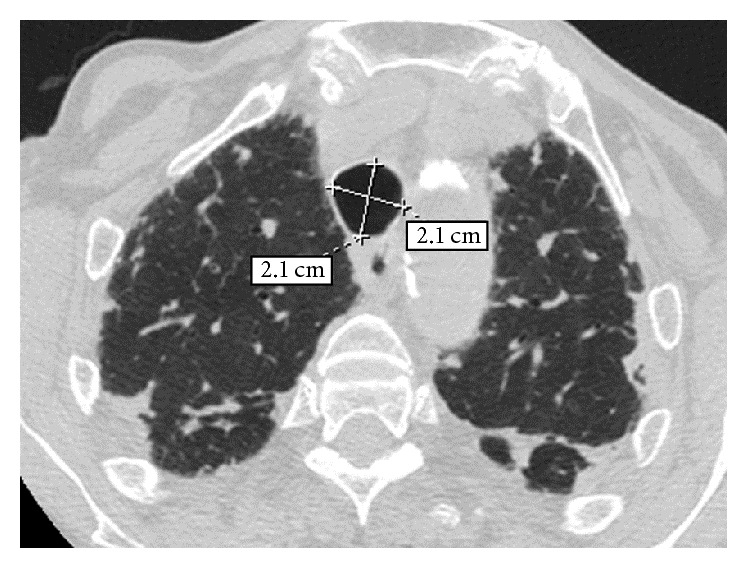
High-resolution computed tomography (HRCT) of the chest at the level of the upper lobes. An enlargement of the trachea at the thoracic inlet is observed measuring in both sagittal and axial dimensions 2.1 cm. A subpleural reticular pattern associated with focal thickening of the pleura and of the major fissures bilaterally.
